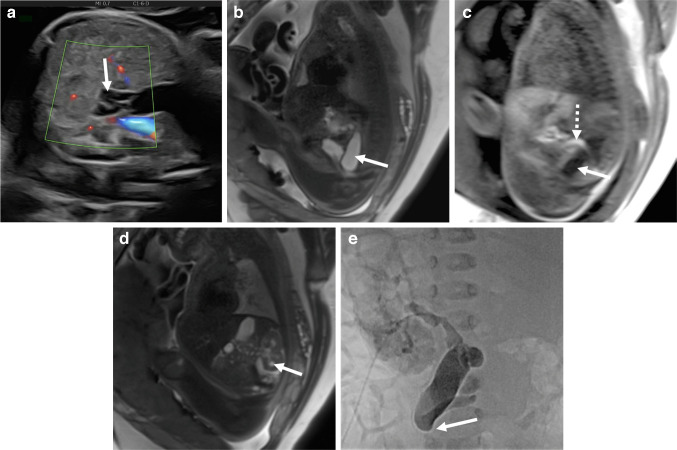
Patient with ureterovesical junction (UVJ) obstruction. a Axial ultrasound of a 29-week gestation male fetus demonstrating a tortuous, tubular anechoic abdominal structure (*arrow*) without internal Doppler flow, consistent with a dilated ureter. b Sagittal Single-Shot Fast Spin Echo (SSFSE) MRI obtained the same day demonstrating a T2-hyperintense tortuous dilated distal ureter (*arrow*). c Sagittal T1 MRI showing T1-hypointense signal within the tubular structure (*arrow*) confirming this to be the ureter, not bowel. Note the T1-hyperintense signal within the adjacent bowel loops (*dotted*
